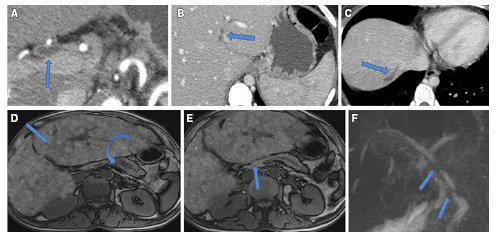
AIP triphasic CT scan (A) arterial (B, C&D) Portal phases: showing thickened wall slightly dilated CHD (Arrow at A), beaded IHBD (Arrow at B), isolated subcapsular IHBD (Arrow at C). Another AIP patient MRI: (D) Biliary cirrhosis (Arrow) with cystic distal pancreatic duct dilatation (Curved arrow), (E) Proximal pancreatic duct stricture (Arrow)& (F) MRCP showing multisegmented CBD stricture. (Arrow).
